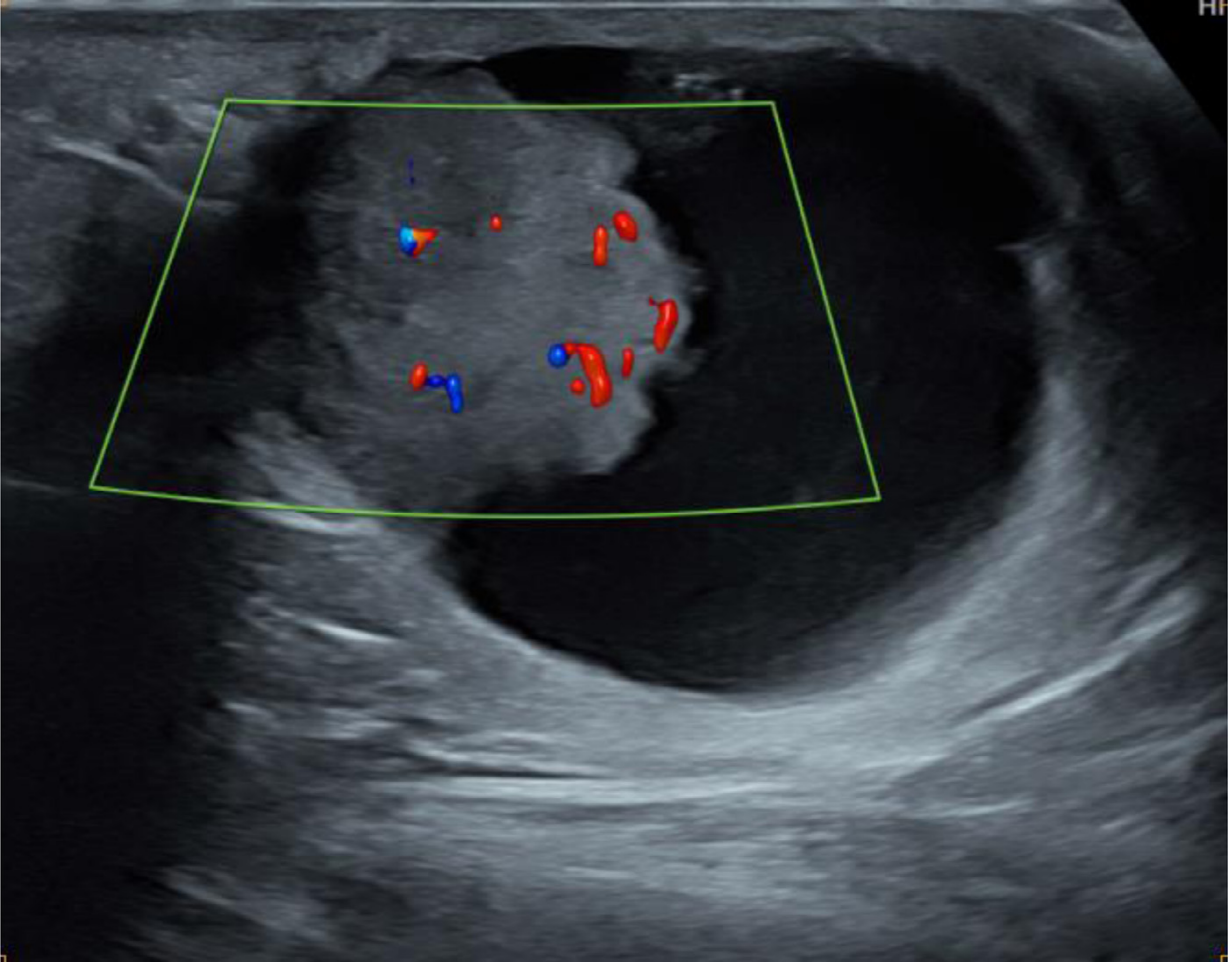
Ultrasound of the left axilla showing Doppler vascularization of the tissue component.
